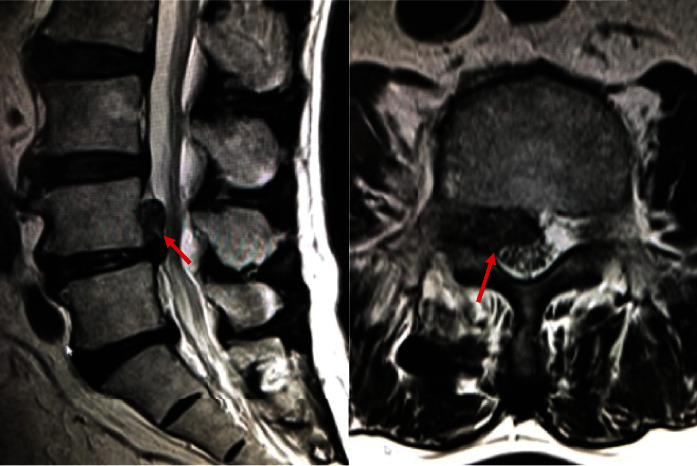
Postdiscogram MR images showed herniated fragment migrated from neural foramen to cranial central canal which was not detected in previous images.
